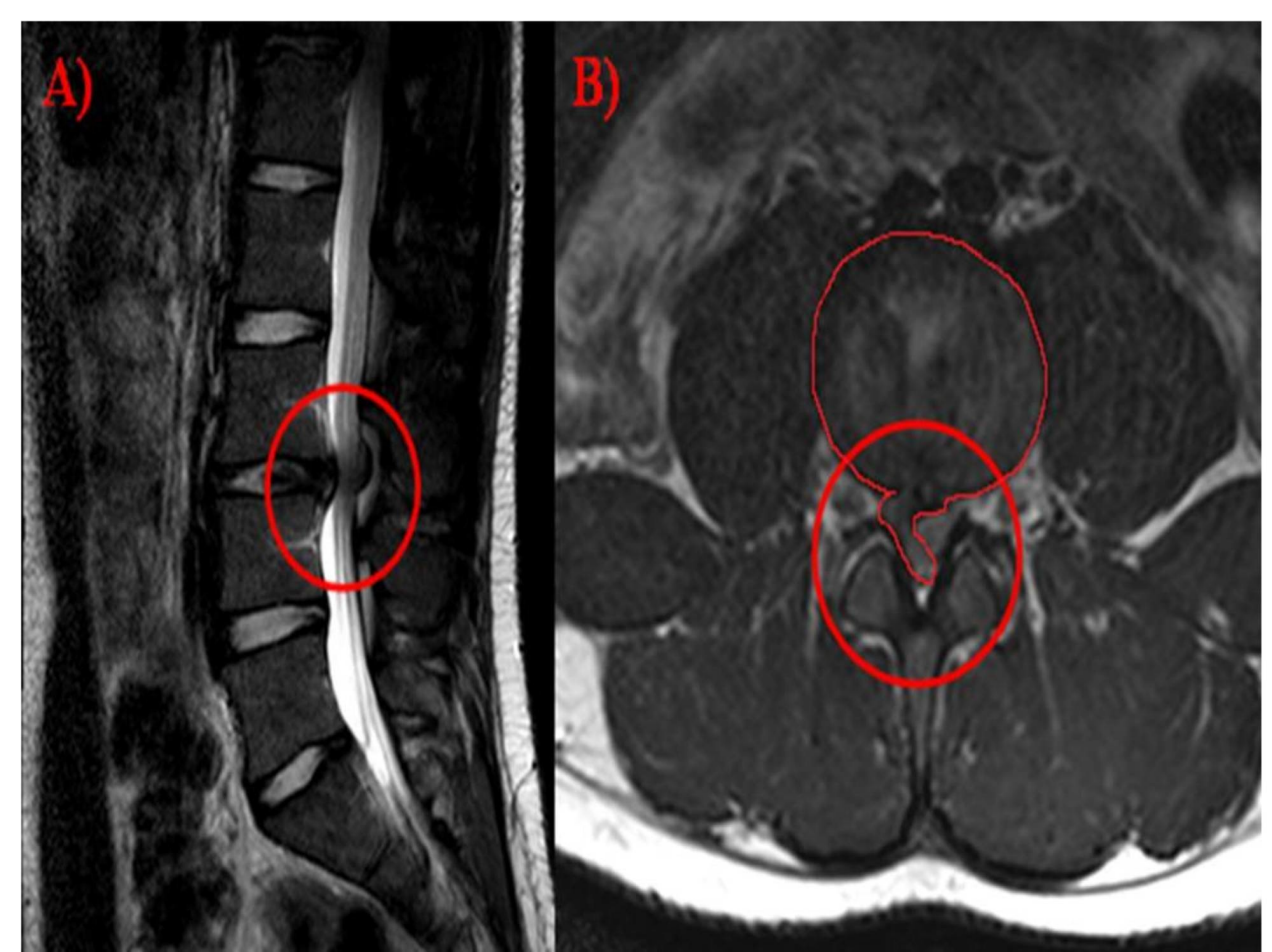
Pre-operative MRI (A) Sagittal T2 MRI showing disc extrusion at the L3-L4 disc space. (B) Axial T2 MRI showing the L3-L4 disc space with disc extrusion to the right and posterior to the thecal sac, resulting in mass effect to the left and compression of the cauda equina on the right. MRI: Magnetic resonance imaging
